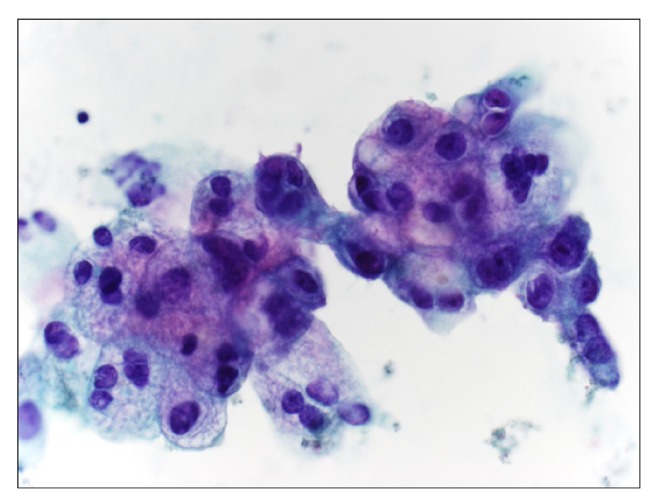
Endoscopic ultrasound (EUS) fine needle biopsy of pancreas revealed adenocarcinoma with mucinous features.
